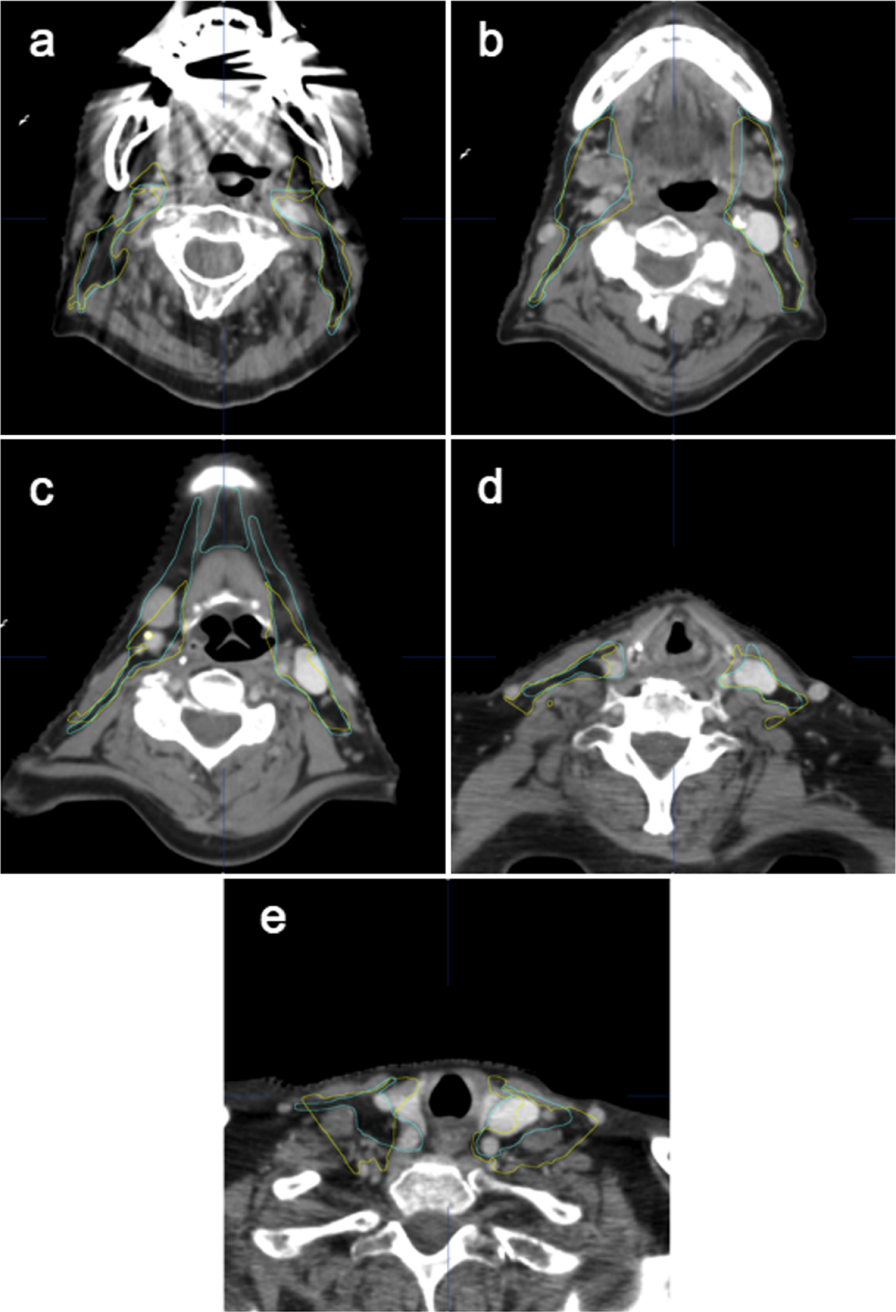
Selected CT slices of patient 12 (N0-stage) with automatic (yellow lines) and manual (blue lines) segmentations. Levels depicted bilaterally in the different panels: a= 2; b and c = 1b and 2; d = 3; e = 4.
